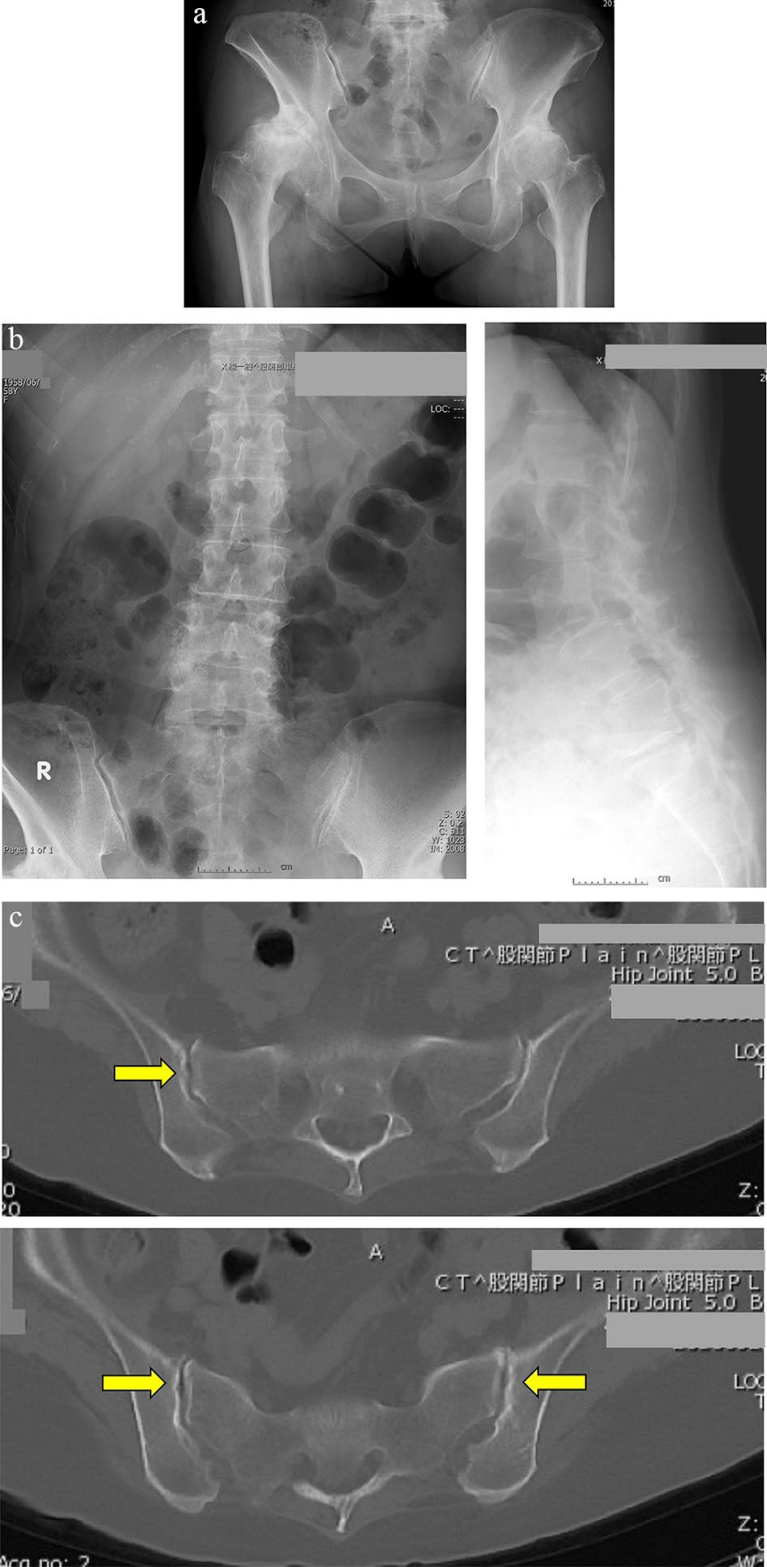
Hip OA secondary to DDH. (a) Pre-operative x-ray. The patient had bilateral terminal-stage OA of the hip joint. The SIJ space differed visibly between right and left sides. (b) Prior to left-side surgery, the patient was diagnosed with a 25% slip of the fourth lumbar vertebra indicative of spondylolisthesis. Scoliosis with a Cobb angle of 7 degrees was also observed. (c) CT imaging showed SIJ irregularities and the presence of vacuum phenomena (yellow arrows). OA: osteoarthritis, DDH: developmental dysplasia of the hip, SIJ: sacroiliac joint, CT: computed tomography.
